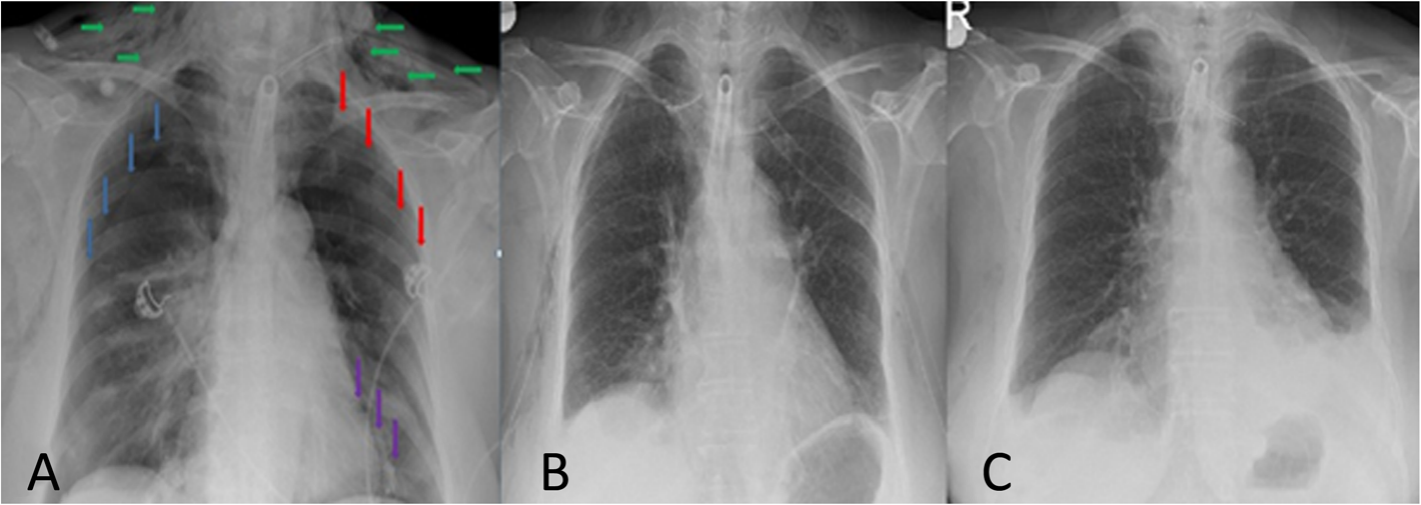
Bilateral pneumothorax, bilateral thorax tube application, and after removal of throax tubes X-Ray. a Bilateral pneumothorax (red and blue arrows) and subcutaneous emphysema (green arrow), b bilateral thorax tube application, and c chest X-ray after removal of throax tubes
